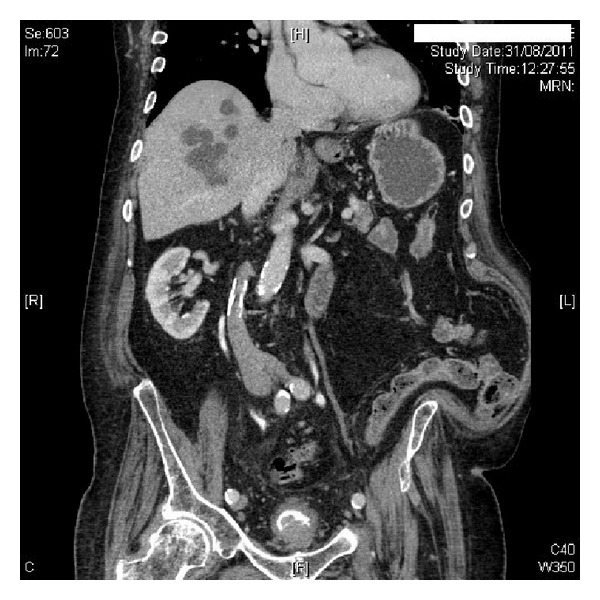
CT urography (coronal view) revealed an irregular mass at the base of urinary bladder.
